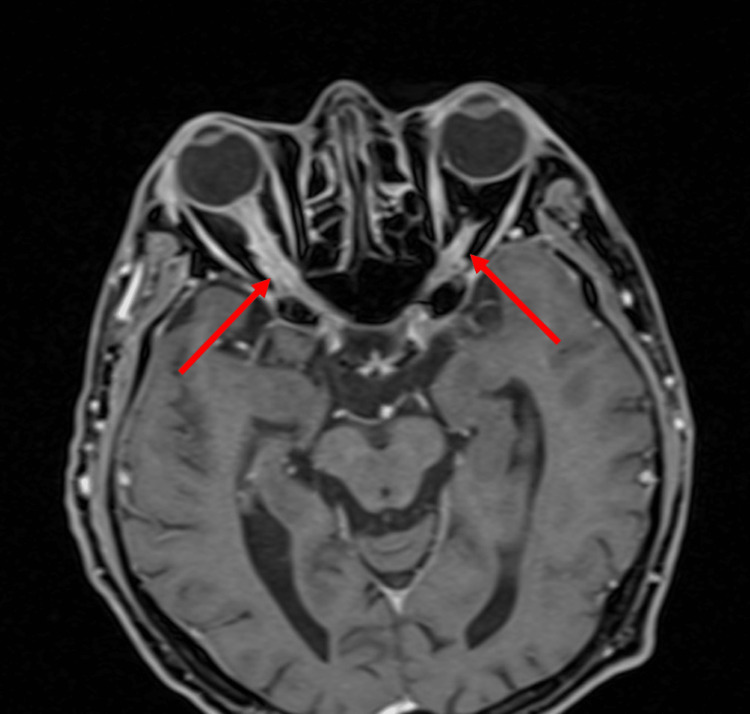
MRI brain T1-weighted VIBE fat-suppressed axial view. Red arrows show optic nerve enhancement involving the entire length of the intra-orbital optic nerve. The classical "tram-track” sign can be appreciated here. VIBE: Volumetric interpolated breath-hold examination.
